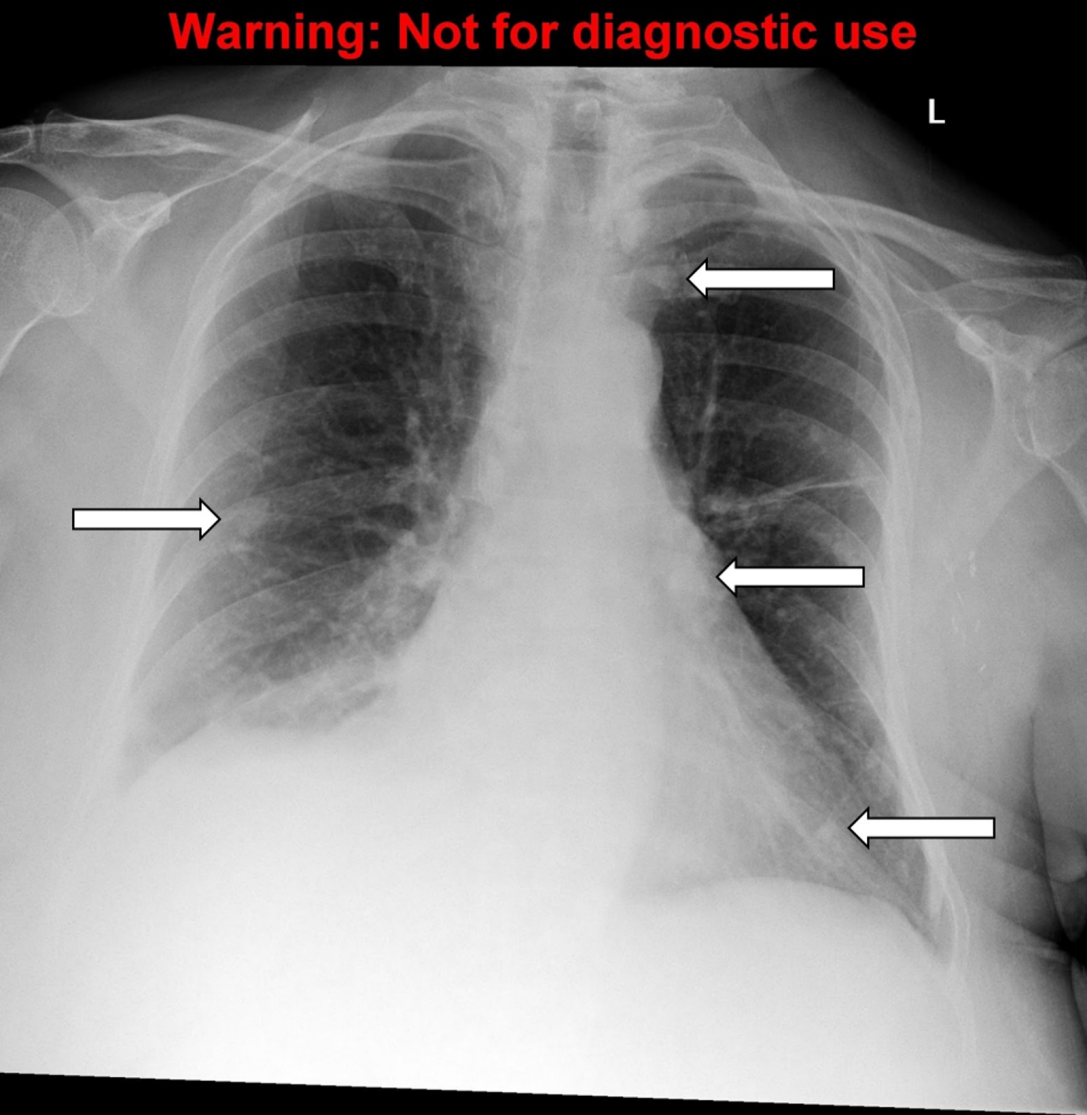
The chest X-ray, anteroposterior (AP) view, demonstrating bone metastasis. Source: A Dixit
